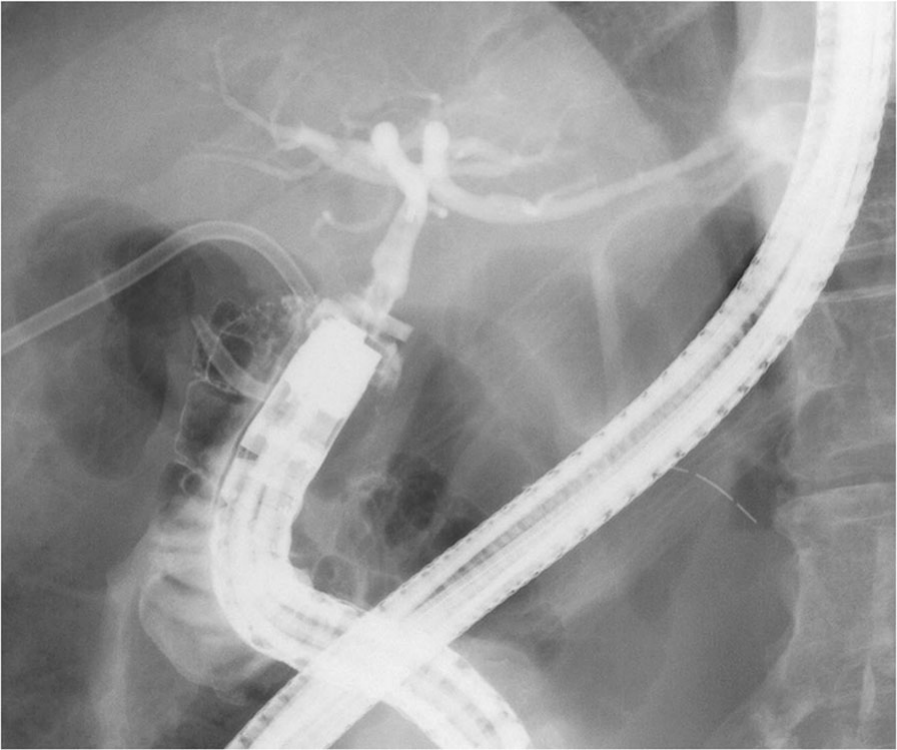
Endoscopic contrast radiography. Only the anterior segmental branch and left branch were visualized; the posterior segmental branch was not visualized
